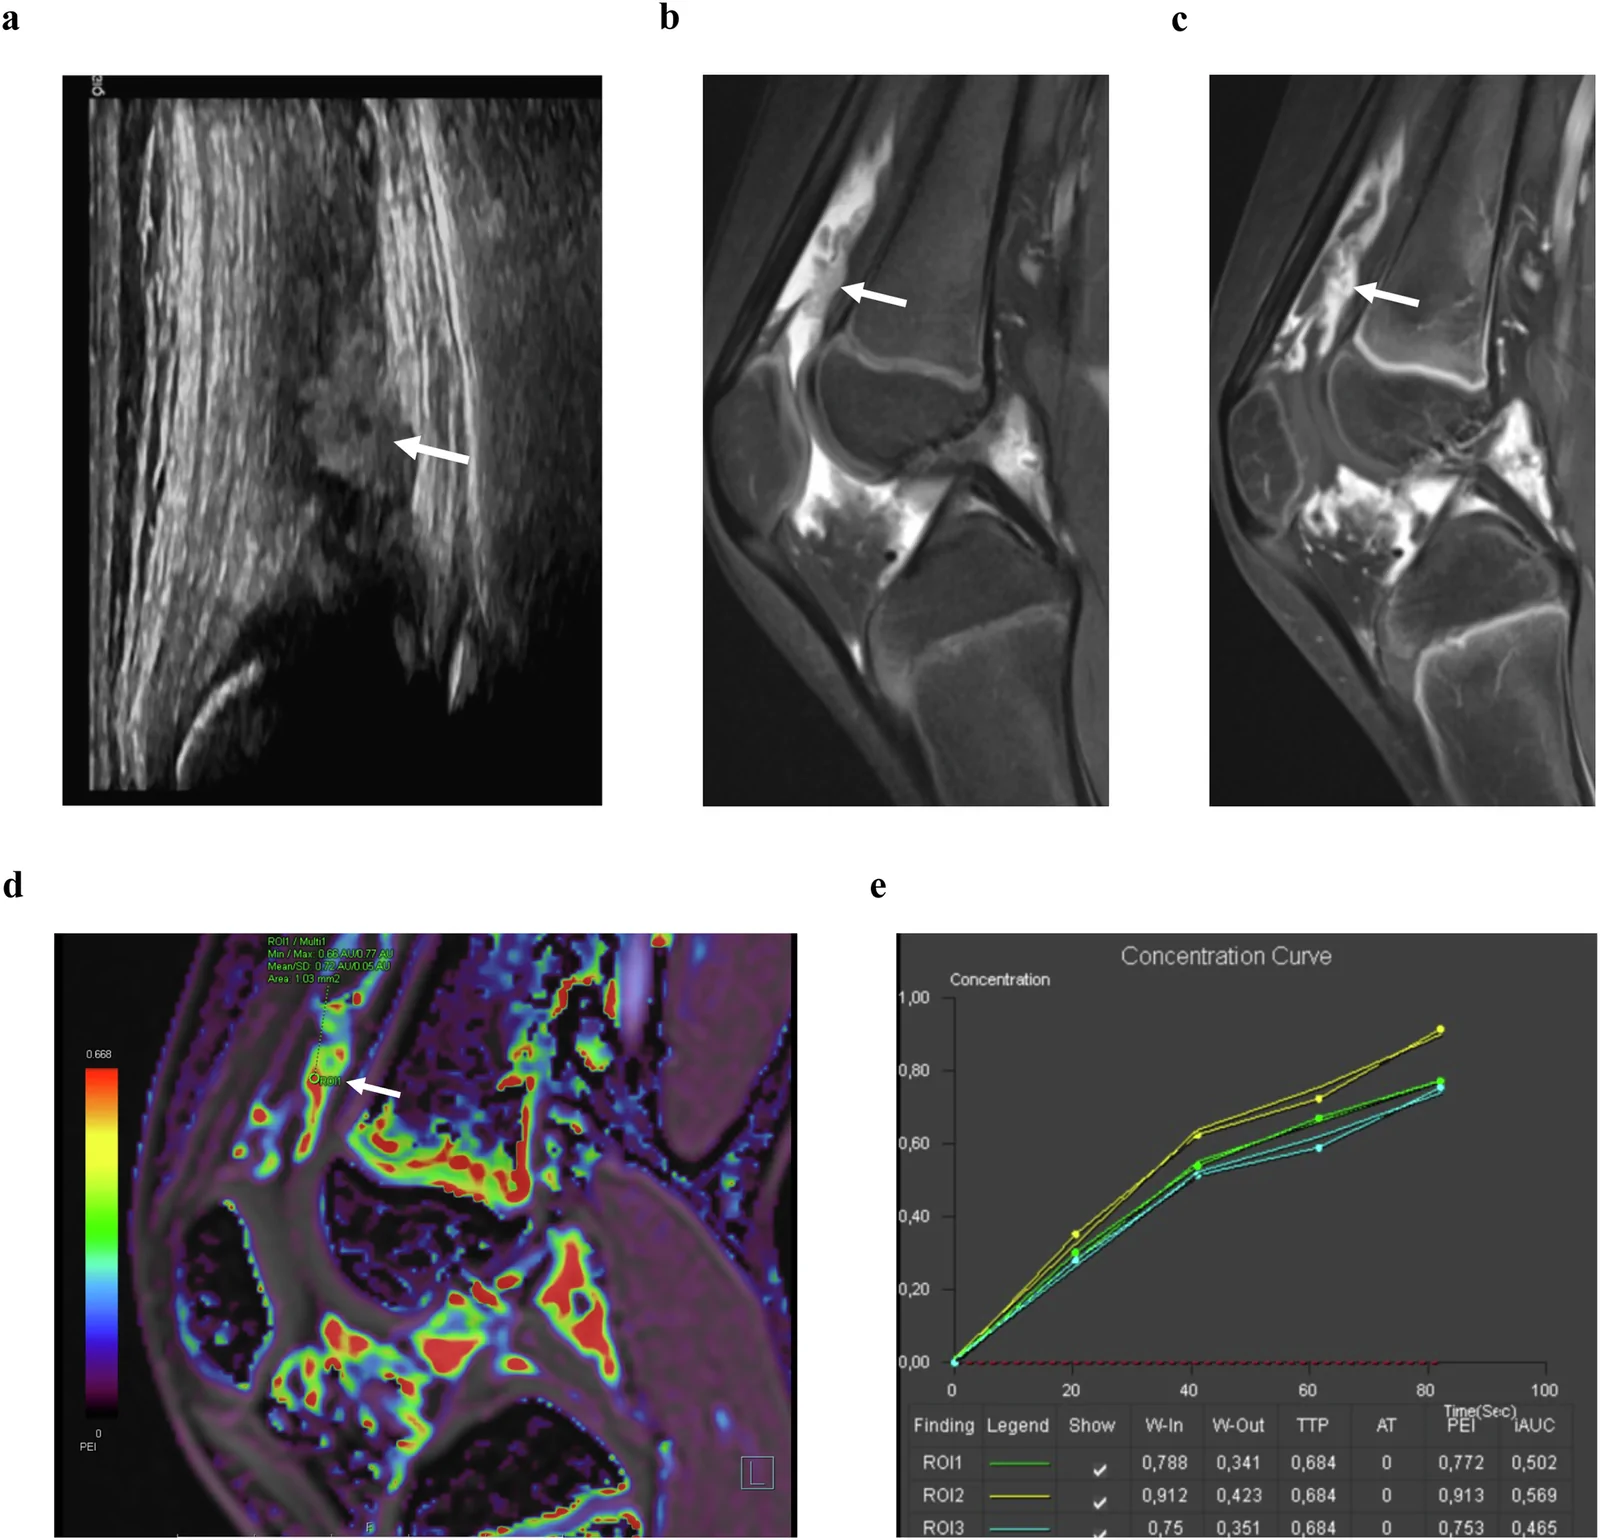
CEMRI in a 7-year-old girl with JIA (same patient as Fig. 1). a B-mode, sagittal view showing thickened synovium (arrow). b MRI PD FS image and c T1 TSE FS postcontrast image, same sagittal plane, showing thickened and enhancing synovium (arrow). d Syngo.via® colour peak enhancement intensity (PEI) map with a region of interest (ROI) placed within the enhancing synovium, in the same areas as for CEUS. e Three dynamic enhancement curves (0–100 s), representing perfusion in 3 different ROIs; concentration curves display the concentration of the contrast agent in the *y*-axis along time in the *x*-axis
